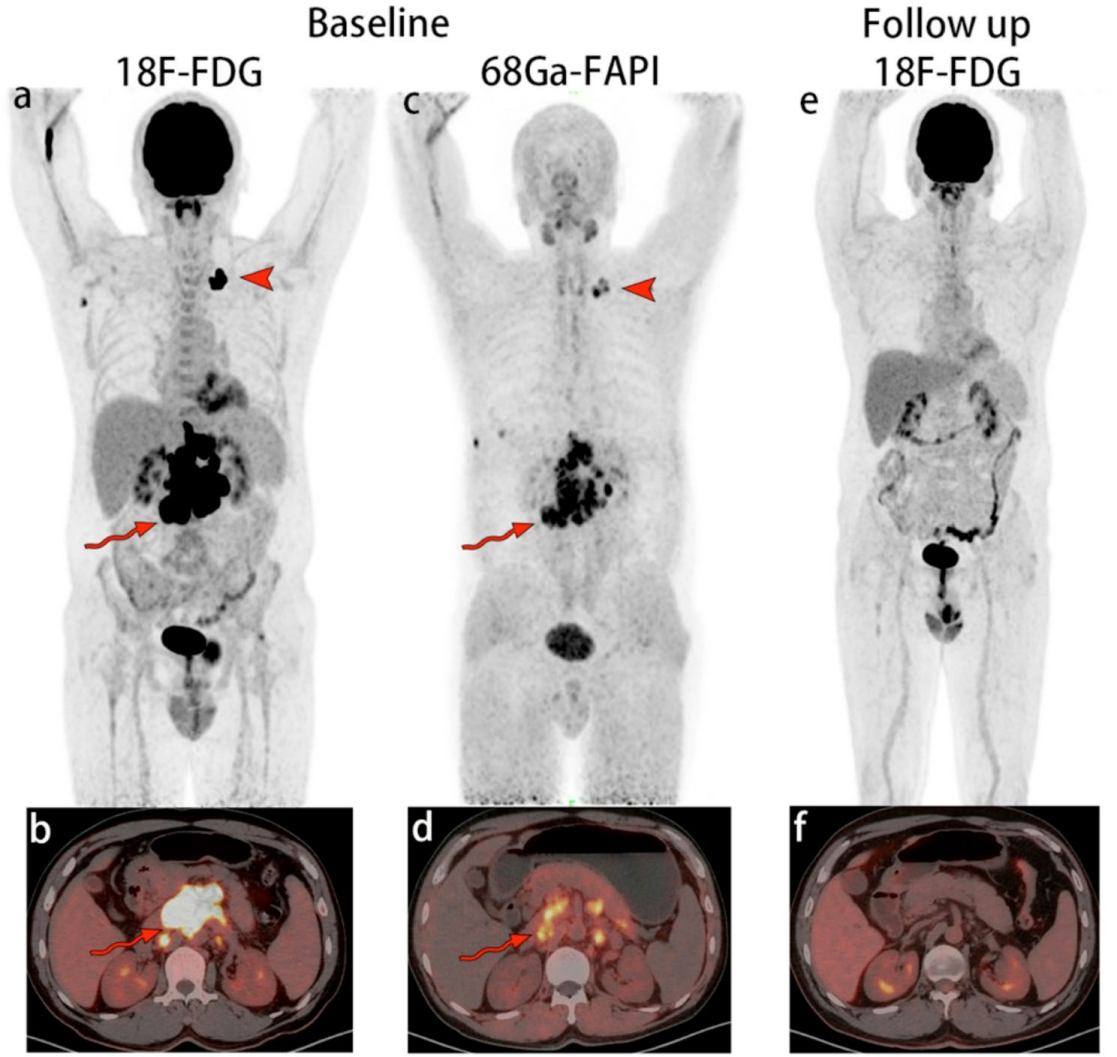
Images of a participant with aggressive large B-cell lymphoma confirmed by biopsy. Received 4 cycles of CHOP chemotherapy. The maximum intensity projection (MIP) image and fusion image of baseline ^18^F-FDG PET/CT showed increased radiotracer uptake of lymph nodes in the abdomen and mediastinum (a, b, curved arrows/arrowhead, SUVmax = 21.0). These lesions were also detected on ^68^Ga-FAPI PET/CT (c, d, curved arrows/arrowhead, SUVmax = 8.0). After 4 cycles of CHOP chemotherapy. A CMR metabolic response were assessed by follow-up ^18^F-FDG PET/CT (e, f. ^18^F-FDG = fluorine 18 (^18^F) labeled fluorodeoxyglucose; ^68^Ga-FAPI = gallium 68 (^68^Ga) labeled fibroblast-activation protein inhibitor; PET/CT = positron emission tomography-computed tomography
